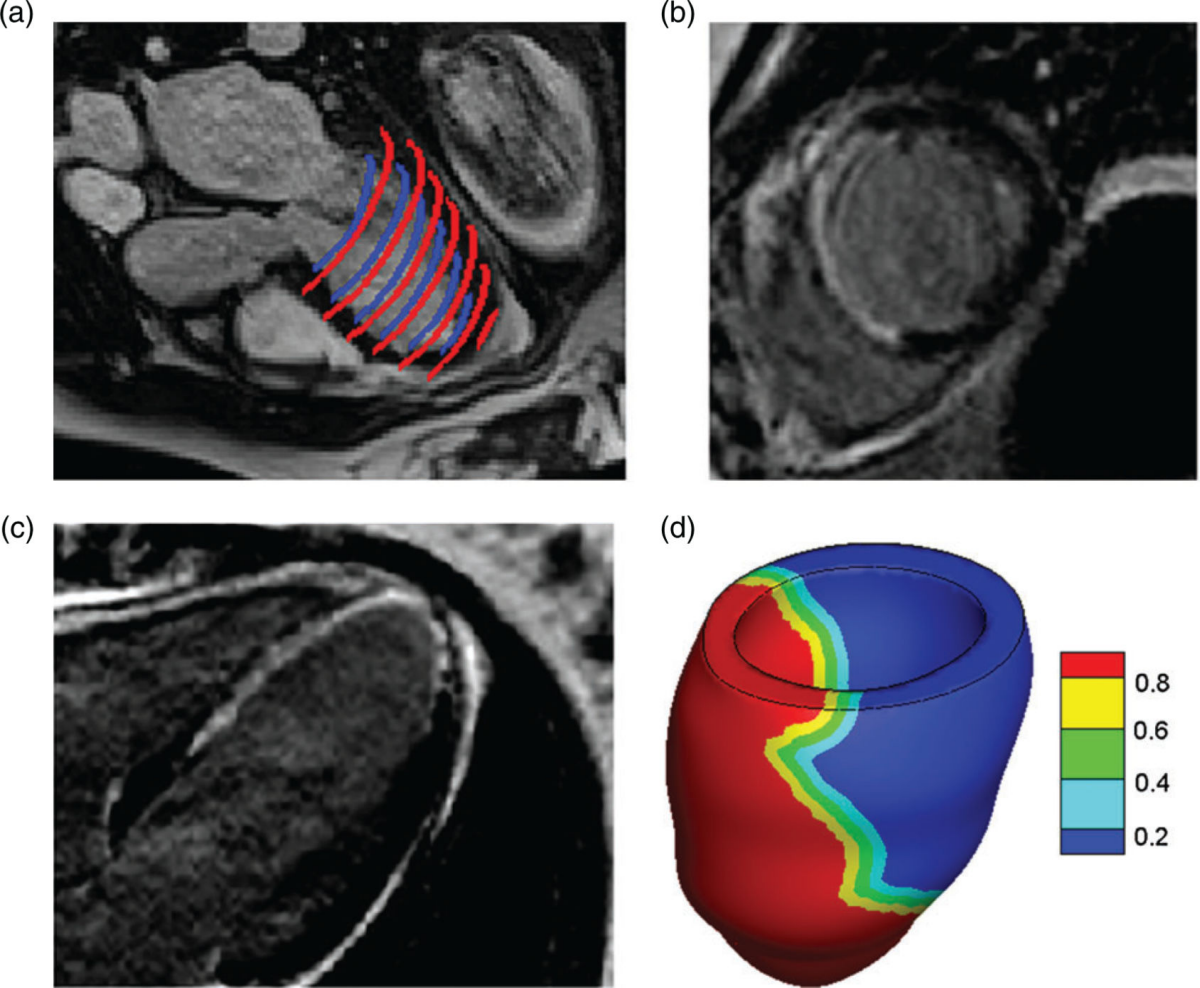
LV geometry reconstruction for the MI patient: (a) endocardial (blue online) and epicardial (red online) boundary segmentations; (b) short-axis LGE MR image slice (the enhanced bright region indicates MI); (c) long-axis LGE MR image slice and (d) reconstructed LV model contoured by the LGE image-based model of MI extent (0: unaffected healthy region; 1: reconstructed infarcted region). A 10-mm thick transition region (border zone) is assumed to lie between the unaffected (blue or right) and infarct (red or left) regions.
